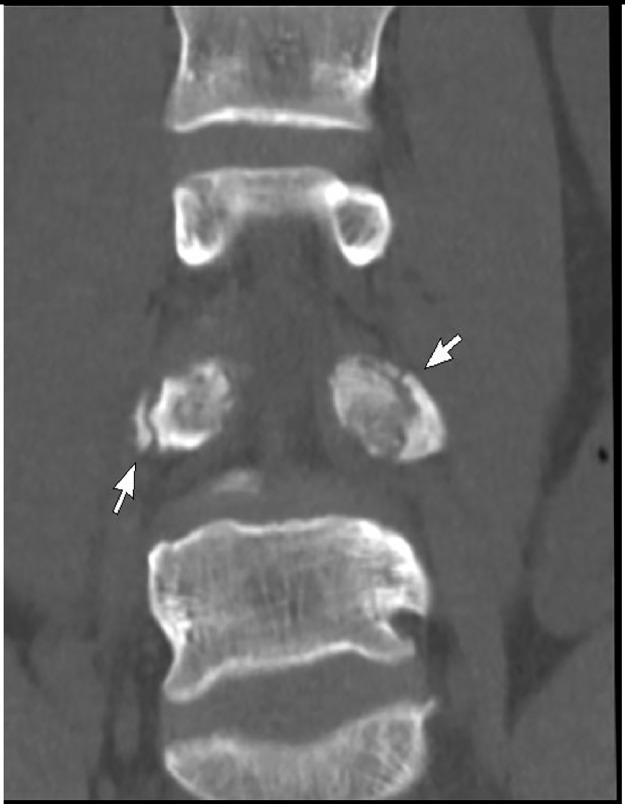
Coronal CT reformats showing bilateral fractures.
